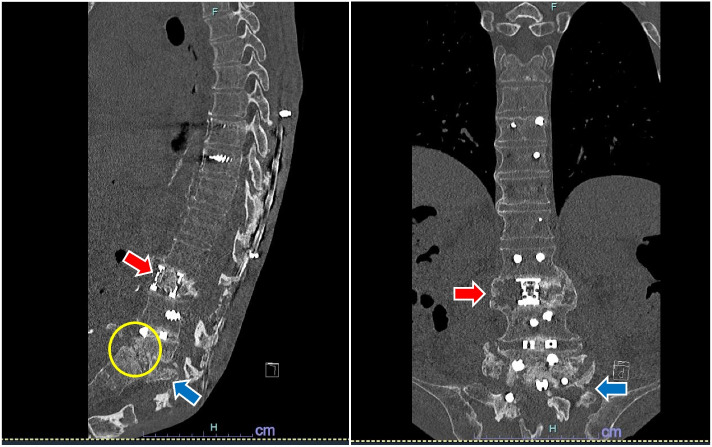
Degree of spinal deformity. Preoperative CT images (sagittal and coronal section). Postoperative posterior spinal fusion from the 9th thoracic vertebra to the 1st sacral vertebra. The 2nd lumbar vertebra (red arrow) and the 5th lumbar vertebra (blue arrow) are highly crushed, and the 4th lumbar vertebra and the 1st sacral vertebra form a false joint (yellow circle)
